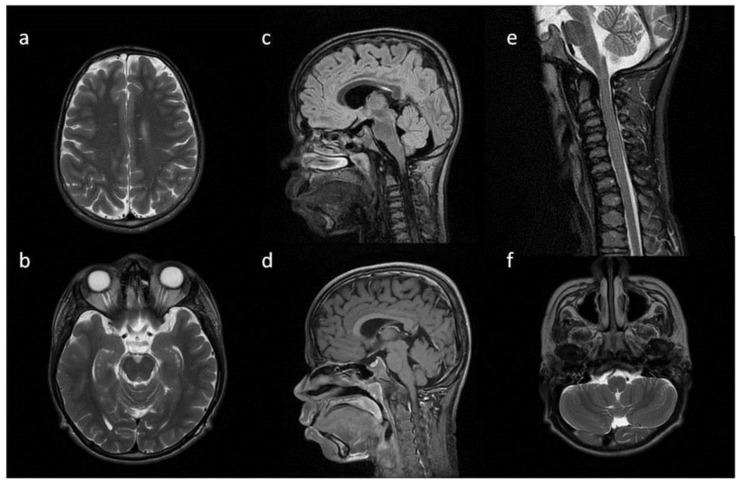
Brain and spine MRI. Patient 5. (a,b,f) axial T2; (c,d) sagittal brain; (c) T2 FLAIR; (d,g) T1; (e) sagittal spine; (e) T2; Findings: (a) enlarged PVS with characteristic location in corpus callosum; (b) optic nerve sheath enlargement; (c,d) flattened anterior border of sella turcica, dysplastic dens; (f) mastoid cells fluid; (e) vertebral and intervertebral discs deformation, cervical spine kyphosis and stenosis.
